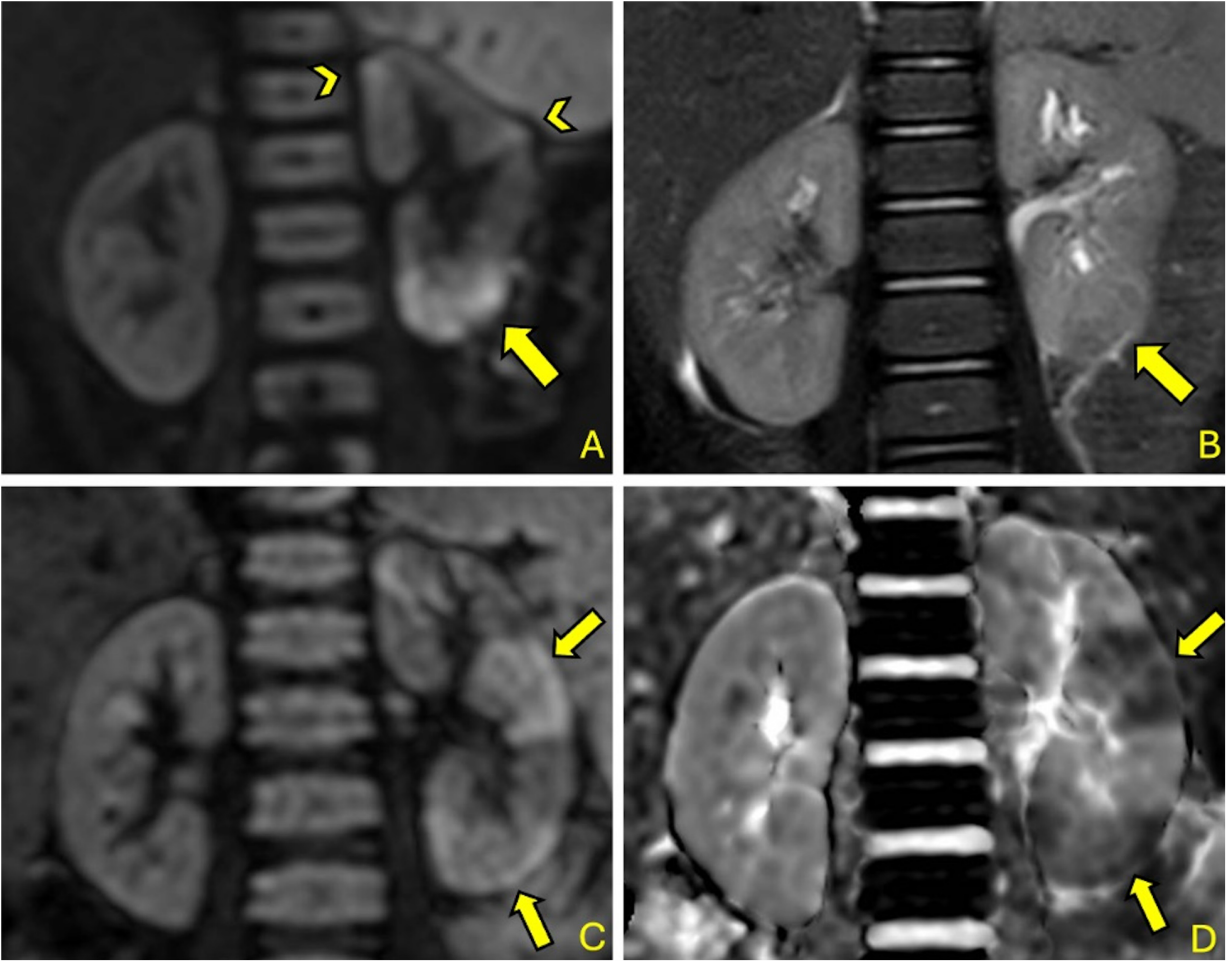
2-year-old girl with fUTI (A&B): DW-MRI (b = 1,000) (A) shows a significant focal area of restricted diffusion at left lower pole (arrow) with corresponding T2 signal alteration (arrow) (B); other smaller foci are pointed by arrowhead (A) 35-month-old boy with fUTI (A&B): DW-MRI (b = 1,000) shows multiple focal areas of restricted diffusion (arrows) (C) with corresponding hypointensities in the ADC map (arrows) (D).
